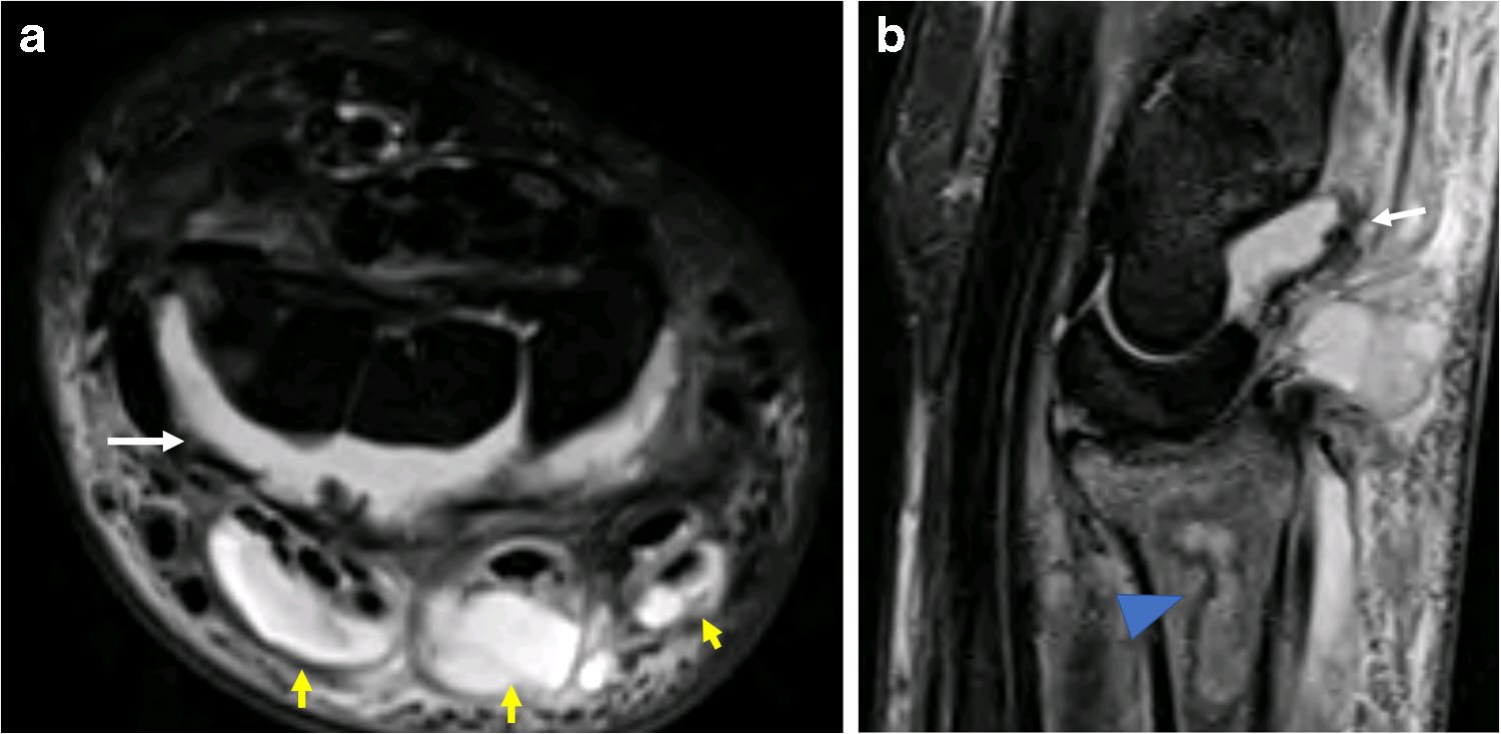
MRI of the right wrist in a 45-year-old man who presented with severe wrist pain, erythema and swelling 5 days after a fluoroscopic-guided wrist corticosteroid injection. Joint aspiration subsequently confirmed septic arthritis. Axial (a) and sagittal (b) STIR images of the right wrist demonstrate large radiocarpal and intercarpal effusions (white arrow), with marked changes of multifocal extensor tenosynovitis (yellow arrows). There is geographic signal abnormality in the distal radius in (b) consistent with osteomyelitis (arrowhead)
